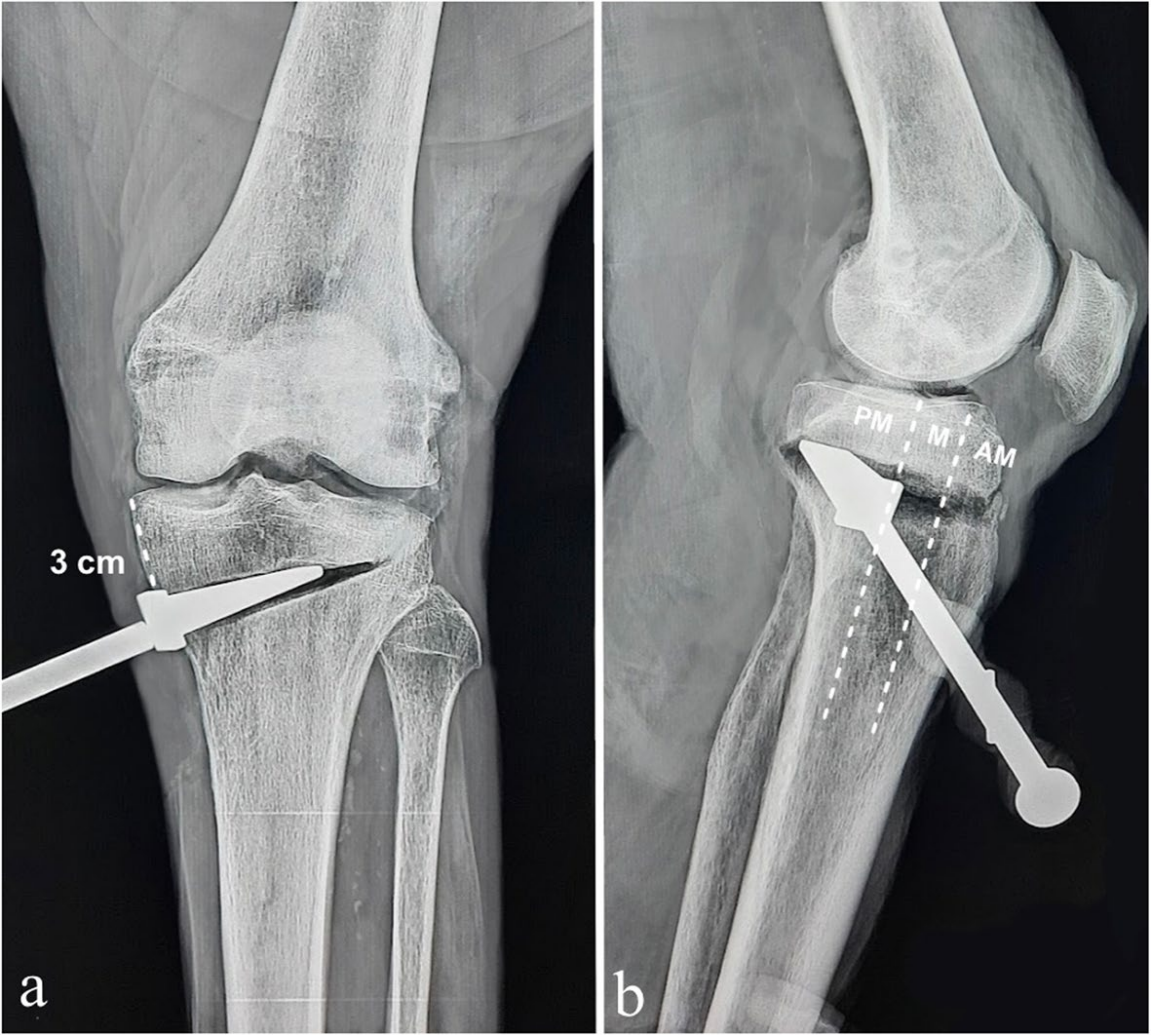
Radiographic control in a left knee, 3 cm case. Anteroposterior view (a) and lateral knee projection (b) with an opening wedge in the PM third location. AM, anteromedial; M, medial; PM, posteromedial
